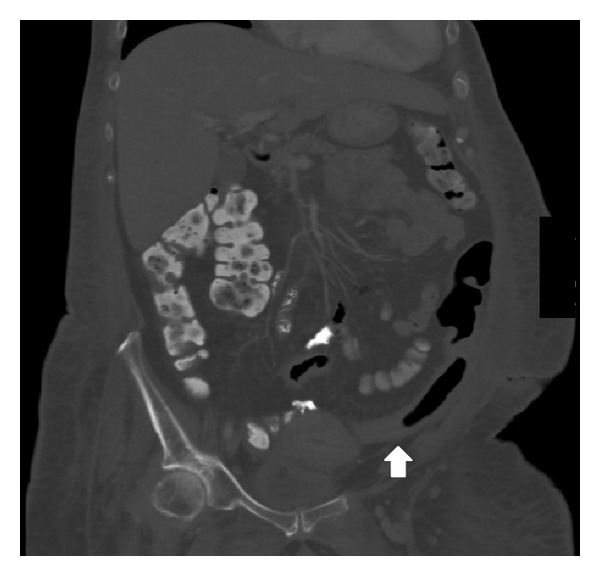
CT of the abdomen/pelvis showing mild wall thickening and an ahaustral appearance of the distal colon with pericolonic fat stranding consistent with colitis.
